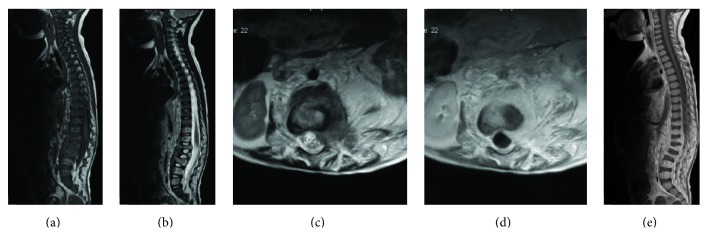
Lesion in the retroperitoneal and paravertebral area and infiltrating vertebrae from T11 to L5. Sagittal MR images show intermediate signal intensity on T1-weighted image (a) and high signal intensity on T2-weighted image (b). Transverse MR image shows high signal intensity on T2-weighted image (c). Transverse and sagittal contrast-enhanced T1-weighted images show homogeneous enhancement of the lesions (d-e).
